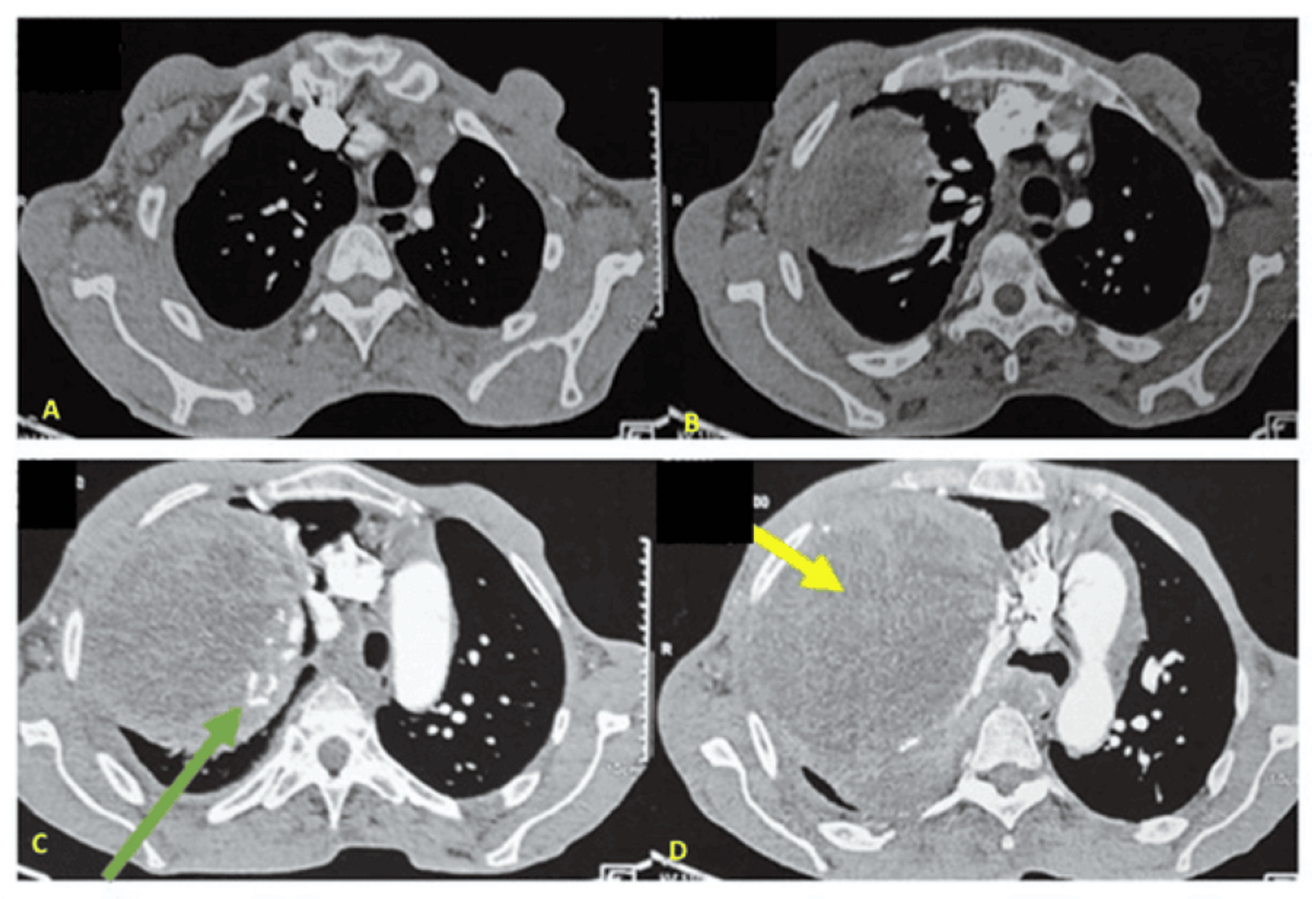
Computed tomography images. (A-D) Voluminous right basithoracic and posterior mediastinal mass (yellow arrow), measuring 184 x 180 mm, heterogeneous with extensive necrosis and calcifications (green arrow) suggestive of tumour recurrence.
